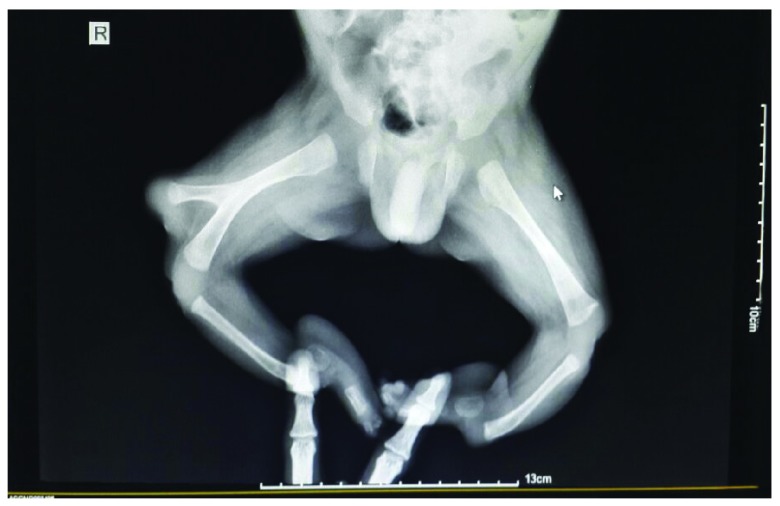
X-ray showing bifid femur with fibular agenesis.
